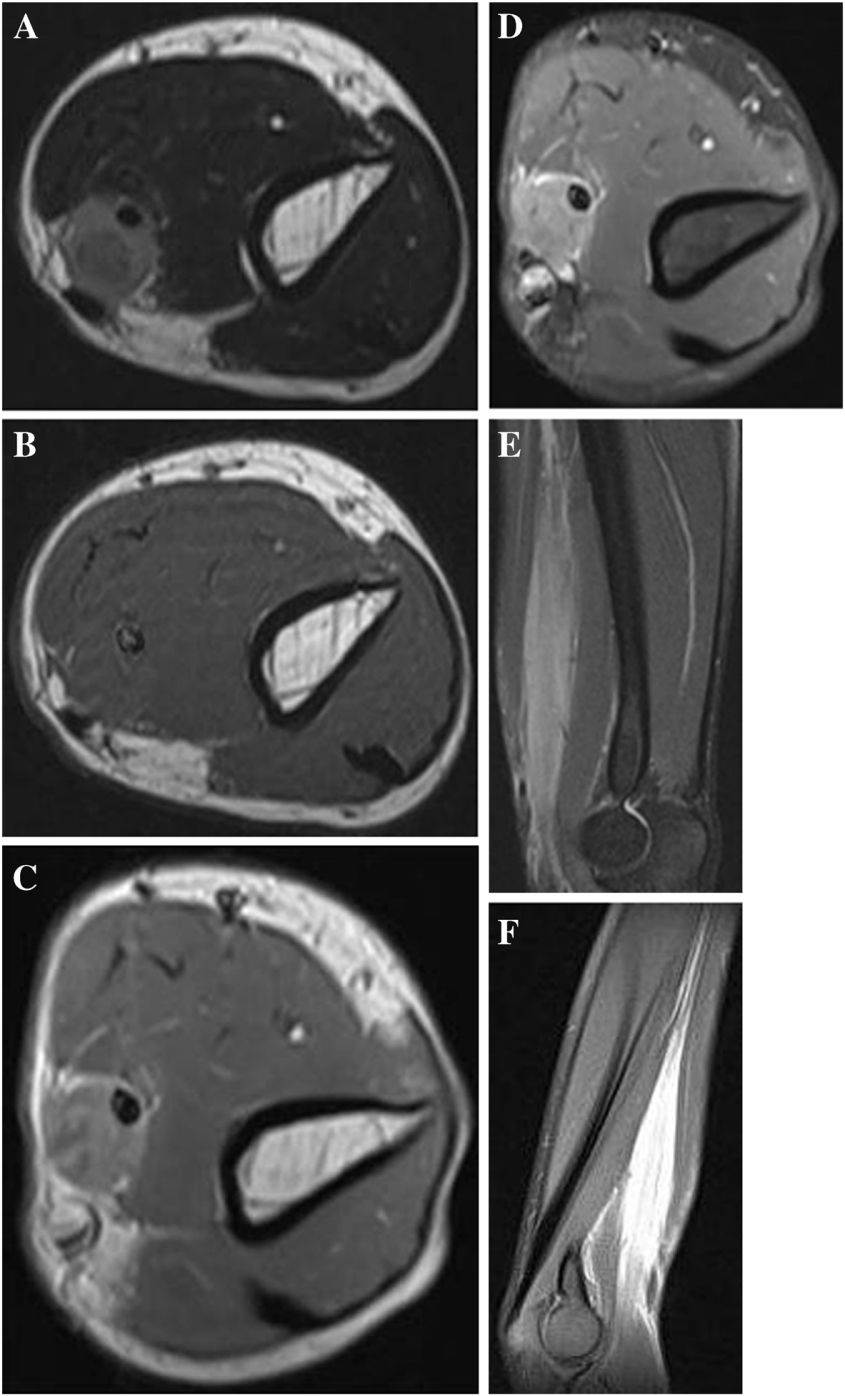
The axial T2-weighted image revealed a homogeneously intermediate signal lesion located within the medial compartments in the left arm. The hypo-intense median nerve is completely encased by the round-shaped mass (A). On the axial T1-weighted image (B) the lesion is isointense to skeletal muscle. The axial enhanced T1-weighted image (C) and axial fat-saturated enhanced T1-weighted image (D) show mildly uniform enhancement and a small area of necrosis. The coronal fat-suppression proton-density fast spin echo image. (E) demonstrates the mass in direct continuity with the median nerve with a fusiform shape. A well-defined lesion is noted on the coronal blade fat-suppression proton-density image (F).
